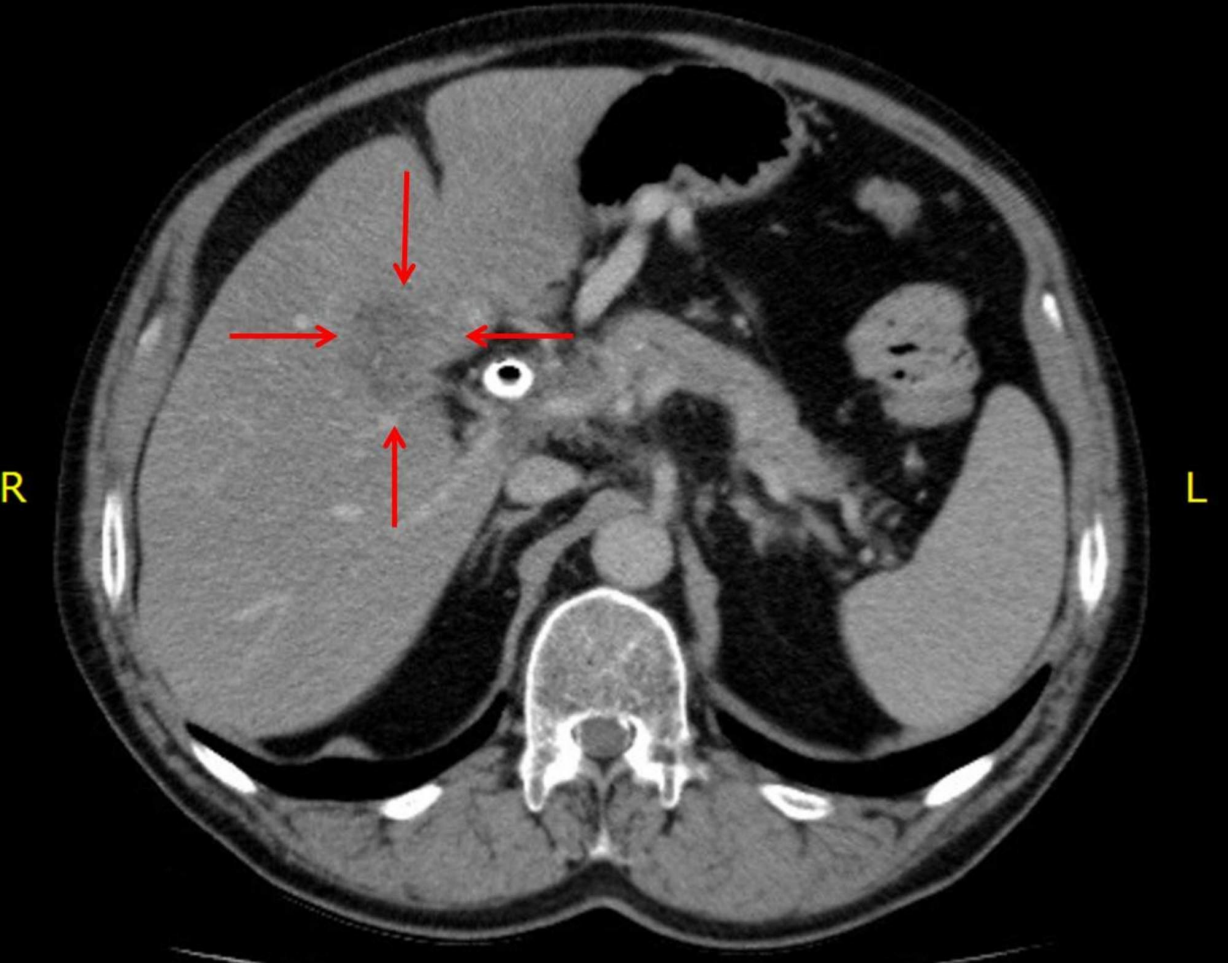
Post-chemotherapy, pre-SBRT CT scan of the abdomen demonstrating a 5.4 cm x 2.8 cm liver metastasis of the cholangiocarcinoma. SBRT, stereotactic body radiotherapy.
